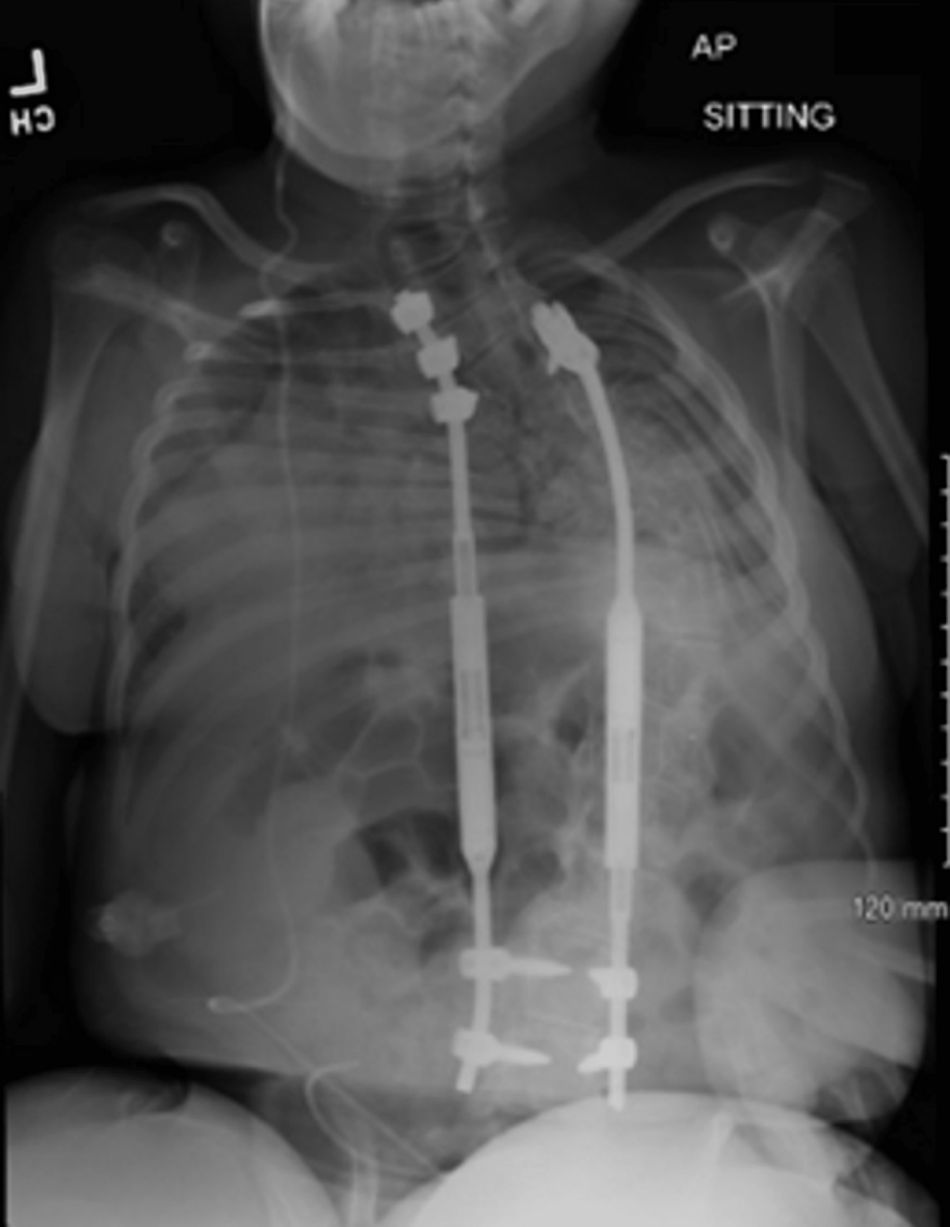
AP plain film performed before conversion surgery from MAGEC rods to fixed cobalt chrome rods.
